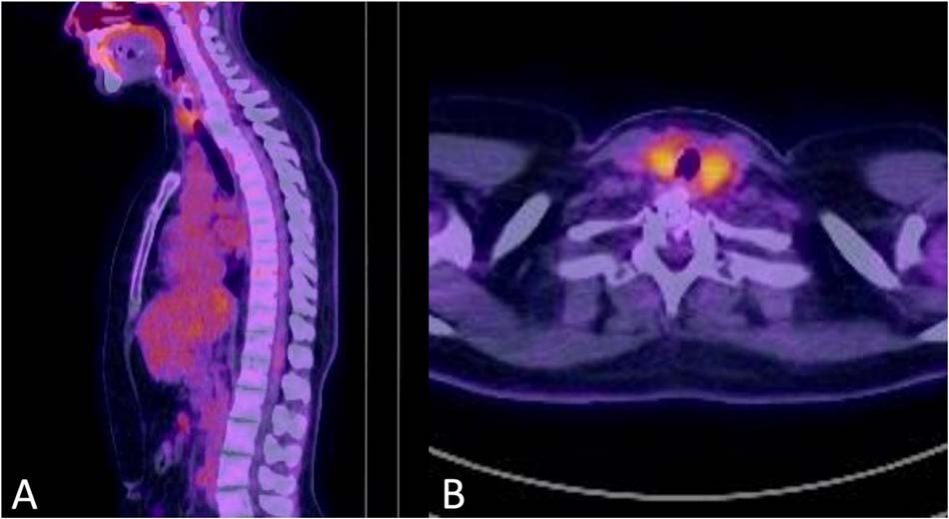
No signs of tumour recurrence at 30-months postoperative follow up PET-CT in July 2022.
